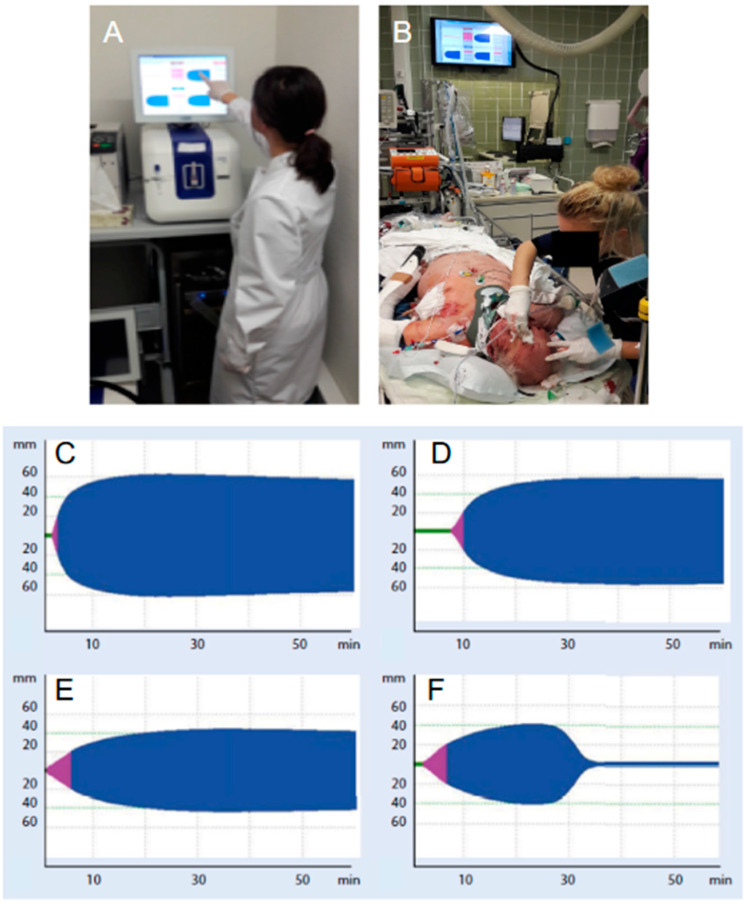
Point-of-care (POC) functional viscoelastic testing in the Emergency Department. The assay can be run at bedside or remote (A) and results be transferred onto a screen in the resuscitation room for rapid clinical decision making (B). Exemplar rotational thromboelastometric traces and interpretation. (C) shows a reference trace, (D) shows a delayed initiation of clotting with prolonged “Clotting time” (CT ↑), (E) shows a reduced clot firmness/stability with reduced amplitude referred to as “Maximal clot firmness” (MCF ↓), and (F) shows a diamond-shaped hyperfibrinolysis with clot breakdown.
